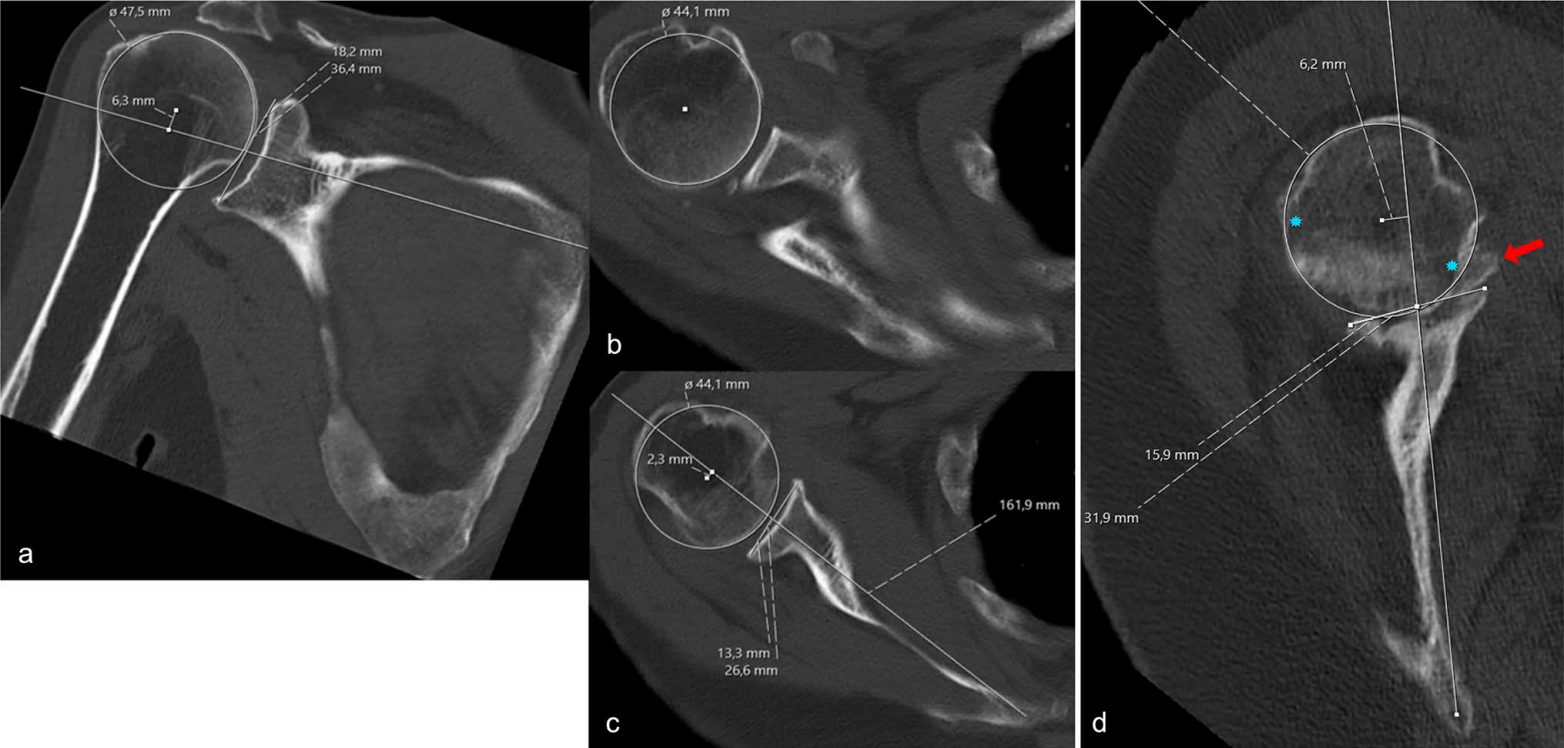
Method for measurement of humeroscapular alignment on A coronal plane and B and C cross–sectional plane computed tomography scans, after reconstruction of the scapular plane in three dimensions. (B) The center of rotation of the humeral head is determined at the widest cross–section of the head and (C) is then translated down to the level of the scapular axis for measurement of subluxation. (D) When higher–grade erosion with partial humeral head collapse and osteophytes are present, measurement is more challenging. Osteophytes (red arrow), whether on the humeral or glenoid side, are disregarded. The center of rotation is determined using the intact outer margins of the humeral joint surface (blue stars) as landmarks [15]
